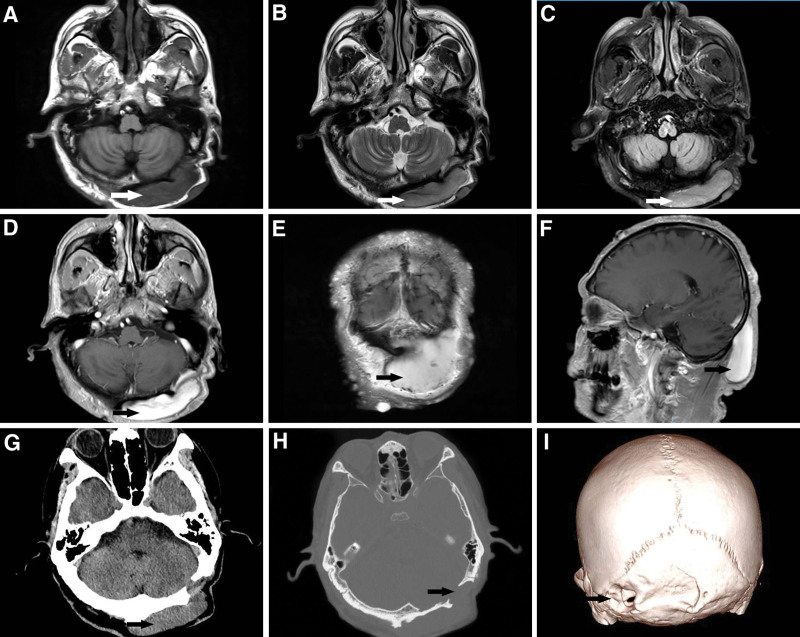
Preoperative MRI scanning for neurofibromatosis type I: T1-weighted, T2-weighted, diffusion-weighted imaging, T1-weighted enhanced axial, coronal, sagittal (A–F); CT plain scan, CT bone window, 3D reconstruction (G–I). CT = computerized tomography, MRI = magnetic resonance imaging.
